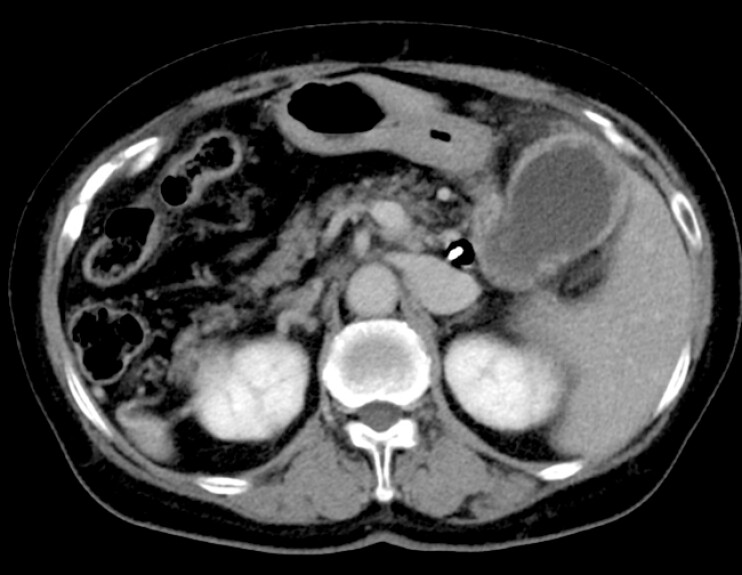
Computed tomographic images obtained before percutaneous transhepatic gallbladder drainage showing a distended gallbladder with wall thickening, consistent with acute cholecystitis.
